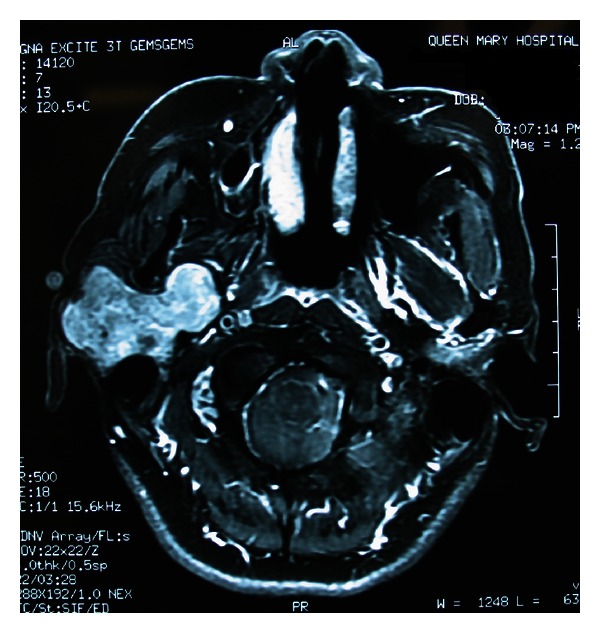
MRI showing right parotid tumour with extension into the deep lobe.
